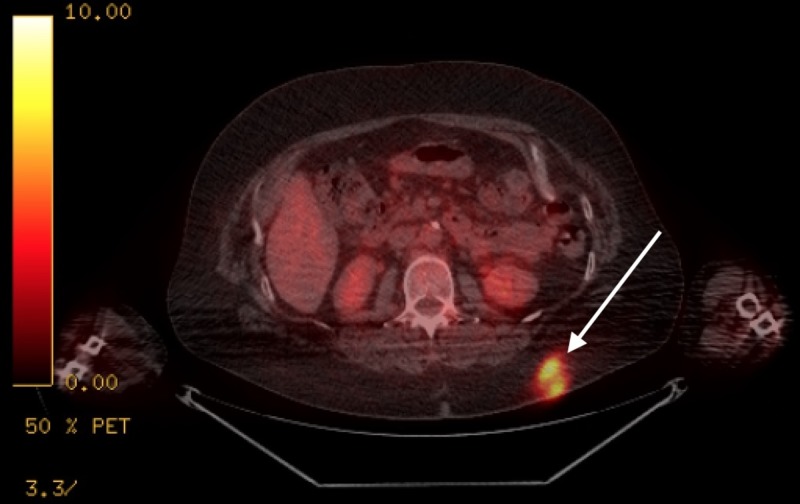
Positron emission tomography-computed tomography (PET/CT) showing hypermetabolic activity in the subcutaneous fat of the upper left buttock area.
